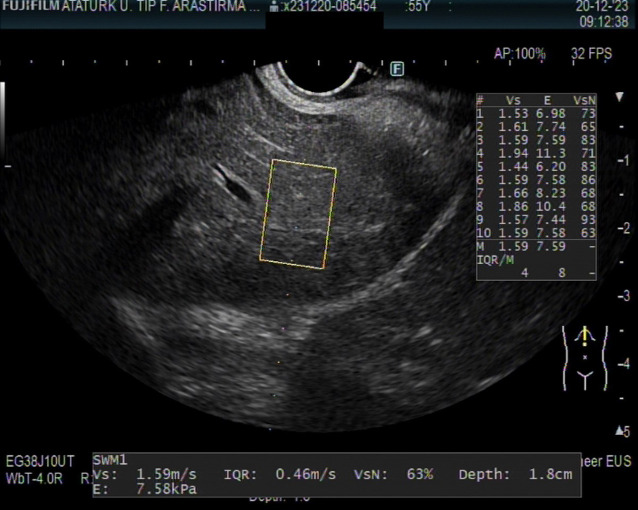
Evaluation of liver stiffness with endoscopic ultrasound shearwave elastography.
